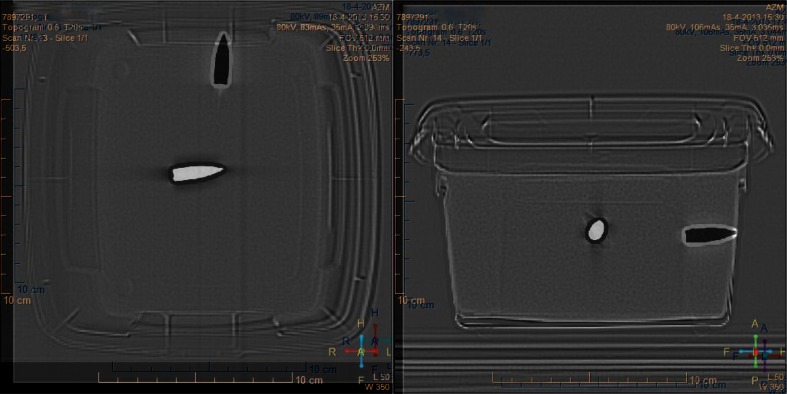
Top view (*left*) and lateral view (*right*) of a CT of gelatine 2. The projectile before exposure, the magnetic field of the MRI is shown in *white*; the position of the projectile after exposure to the MRI is shown in *black*. A clear displacement of the projectile has occurred along the direction of the main axis of the static MRI field in combination with a rotation of the projectile
